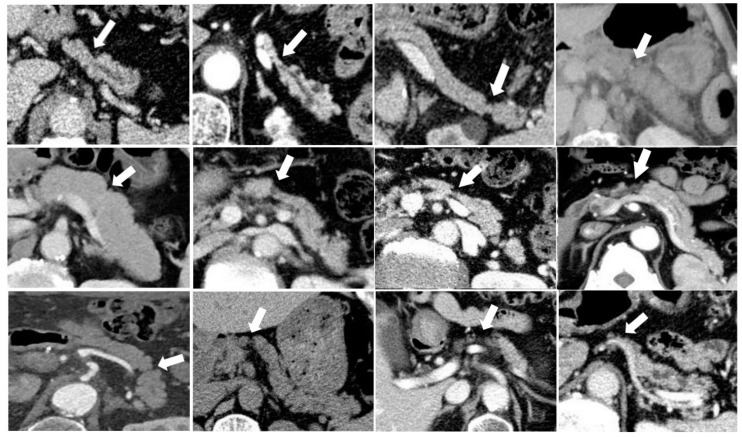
CT images of various types of focal parenchymal atrophy (white arrows).
